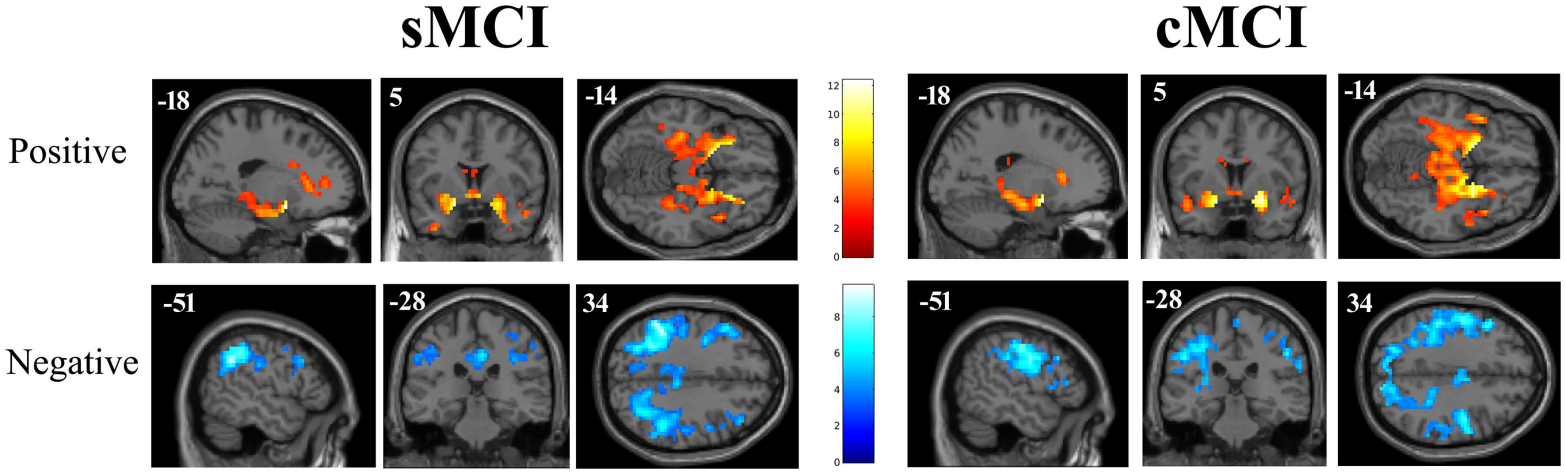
Positive (top) and negative (bottom) functional connectivity networks of the primary olfactory cortex in stable (left) and converter (right) MCI patients are shown in sagittal, coronal, and axial views (neurological convention) overlaid on the standard T1-weighted structural template implemented in SPM12. Results are displayed with a cluster size threshold *k* ≥ 31, corrected at *α* < 0.05. Color bars represent *t*-values.
